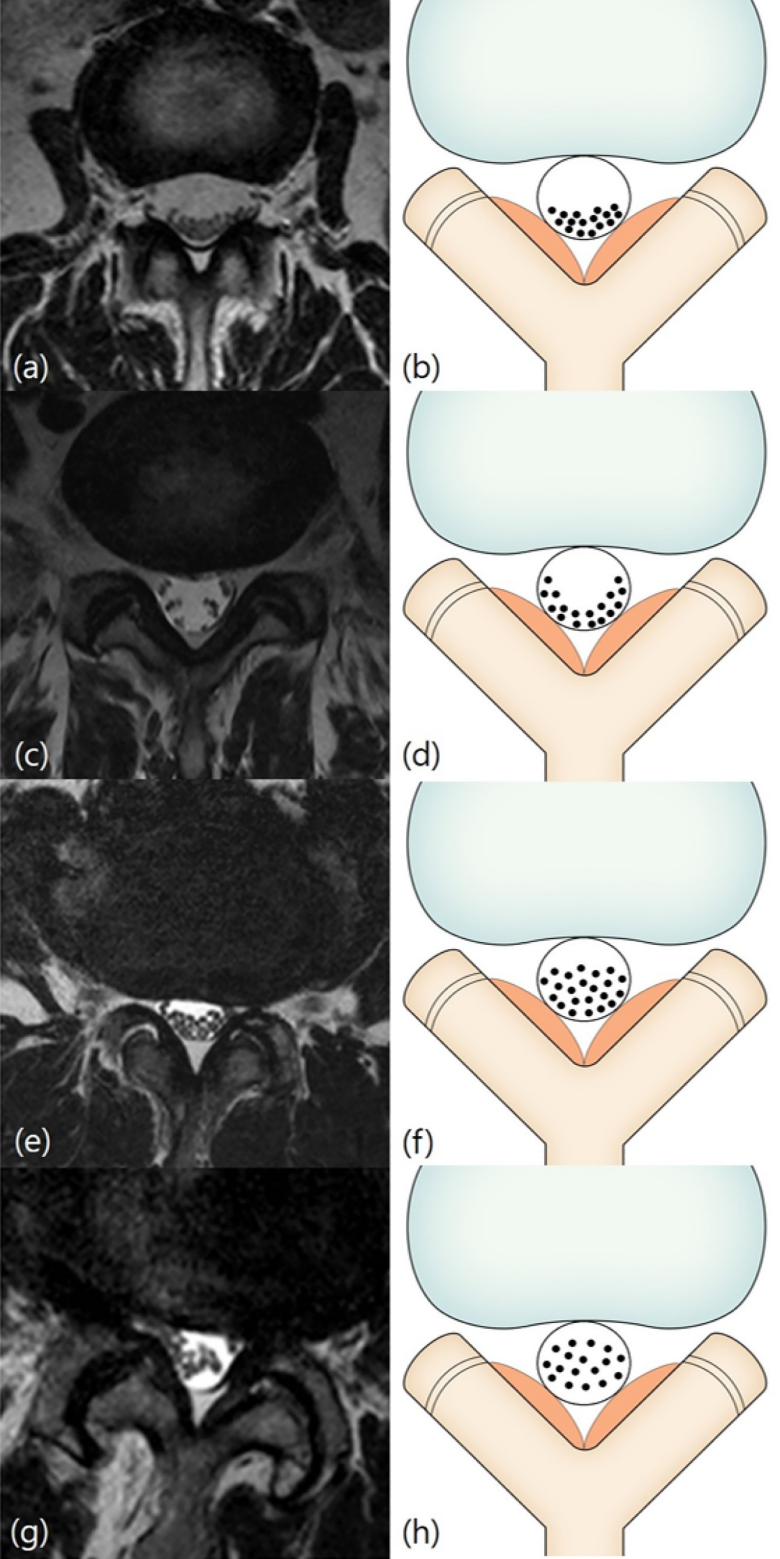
Schizas classification. Grade A (no or minor stenosis). Fig 1A and 1B: Grade A1 (no or minor stenosis). A 59-year-old woman with right lower extremity radiculopathy. T2-weighted axial magnetic resonance image at L2/3 disc level shows the rootlets lying dorsally and occupying less than half of the dural sac area. Fig 1C and 1D: Grade A2 (no or minor stenosis). A 65-year-old man with pain in both buttocks. T2-weighted axial magnetic resonance image at L5/S1 disc level shows the rootlets lying dorsally with a horseshoe configuration. Fig 1E and 1F: Grade A3 (no or minor stenosis). A 71-year-old man with left lower extremity radiculopathy. T2-weighted axial magnetic resonance image at L3/4 disc level shows the rootlets lying dorsally and occupying more than half of the dural sac area. Fig 1G and 1H: Grade A4 (no or minor stenosis). A 77-year-old man with lower back pain. T2-weighted axial magnetic resonance image at L3/4 disc level shows the rootlets lying centrally and occupying the majority of the dural sac area.
